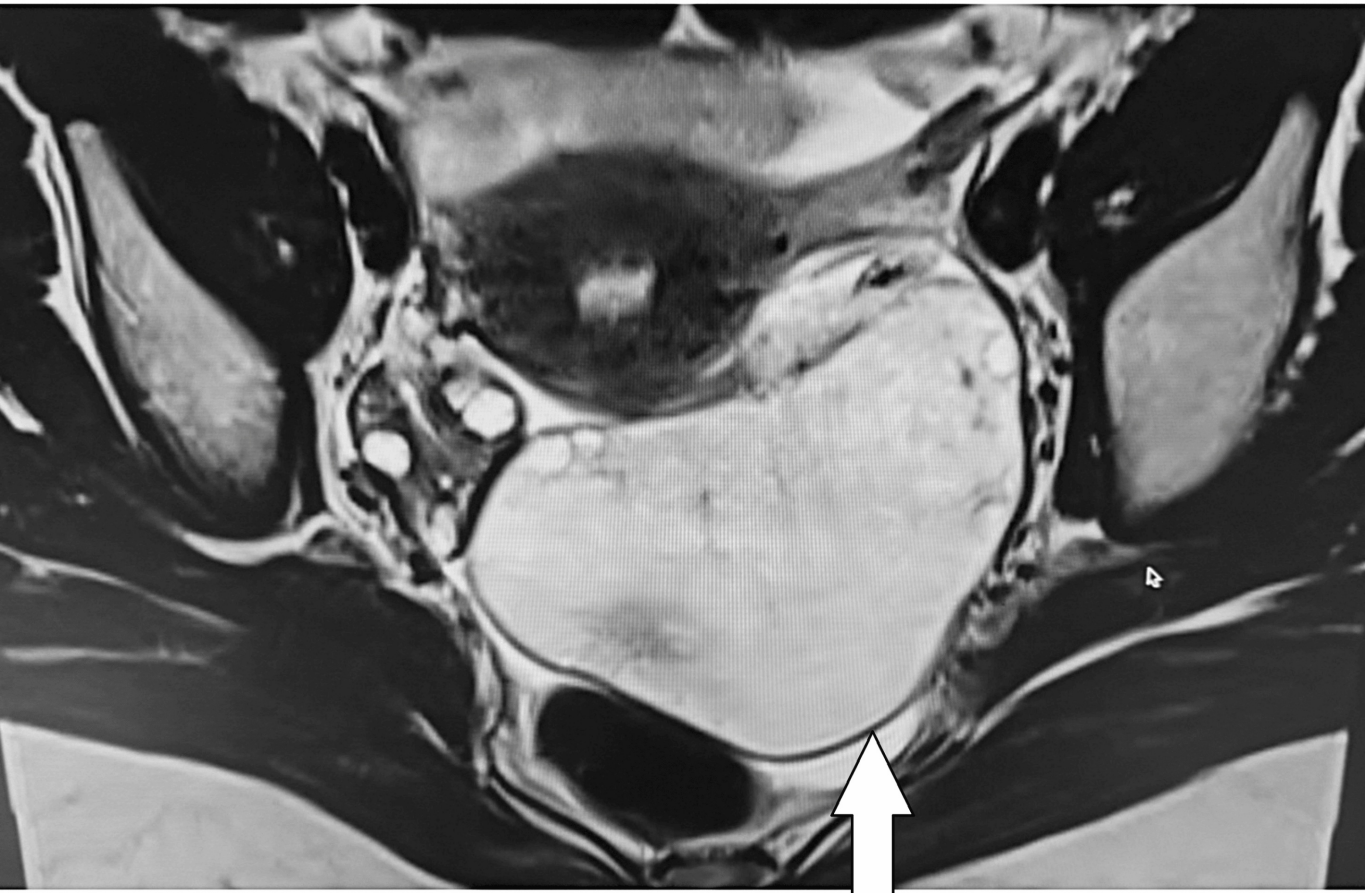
Axial T2-weighted pelvic MRI image showing homogeneously high signal intensity within the enlarged left ovary (white arrow).
